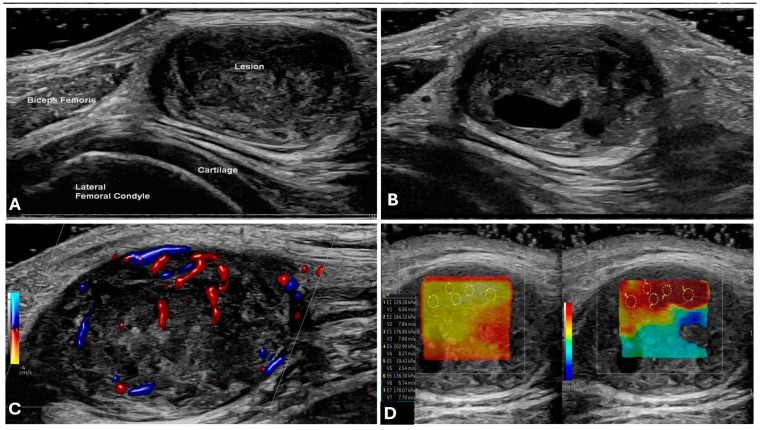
Benign peripheral nerve sheath tumor (schwannoma) of the tibial nerve in a 42 year old woman. Sagittal greyscale ultrasound (A,B), sagittal color Doppler (C), and sagittal elastography (D) images showing a well-circumscribed, heterogenous echogenicity lesion (arrows) with mild organized intrinsic vascularity (thick arrows).
